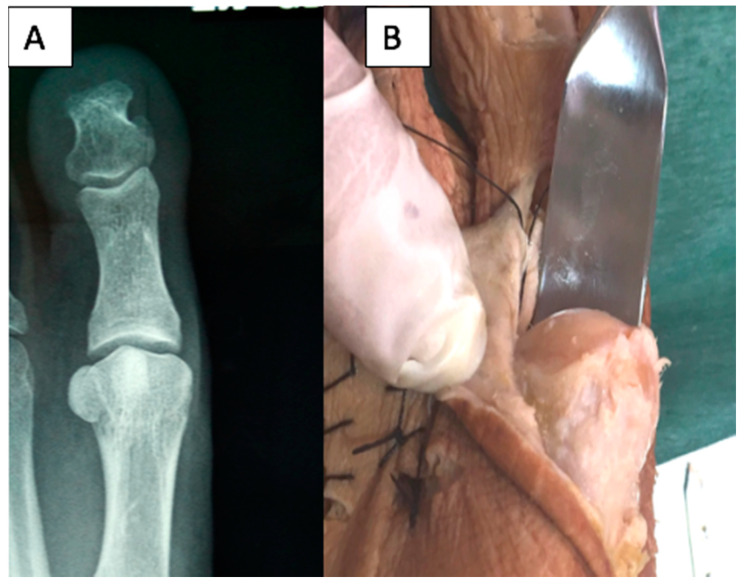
Views of a first metatarsal head showing distortion to appear crest shaped in a radiographic image performed to 30° (A) and after dissection revealing a round shape (B).
